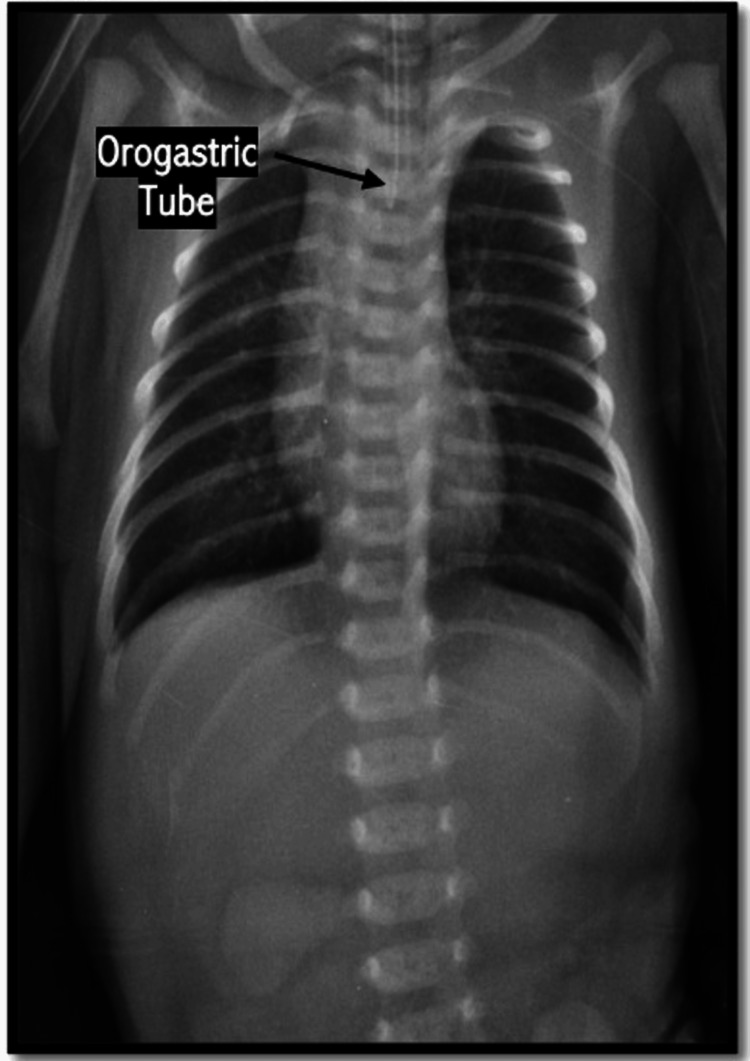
Absence of gastric bubble Thoracoabdominal X-ray showing an orogastric tube coiled at the cervical esophageal level with absence of a gastric bubble. The study was performed in a newborn presenting with sialorrhea and respiratory distress requiring mechanical ventilation after unsuccessful advancement of the orogastric tube. Findings are suggestive of esophageal atresia.
